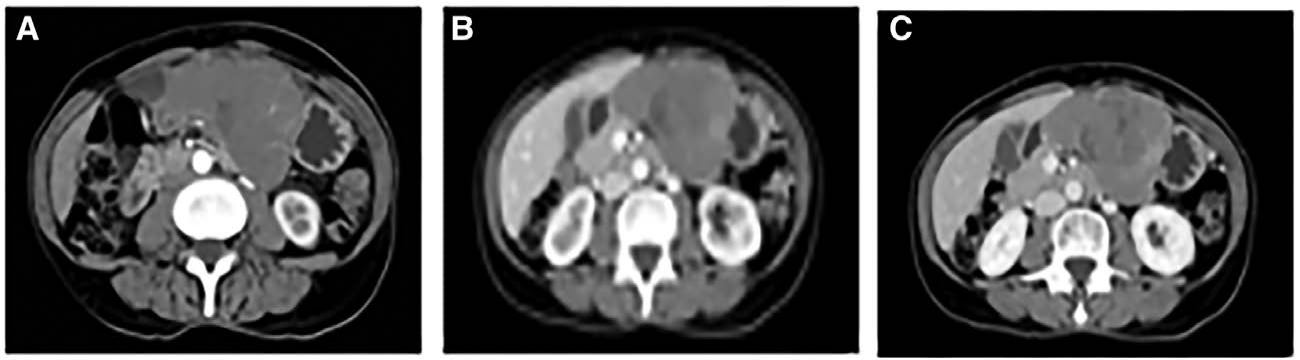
Computed tomography scans of the tumor. (A) Arteria phase. (B) Portal venous phase. (C) Delayed phase.
